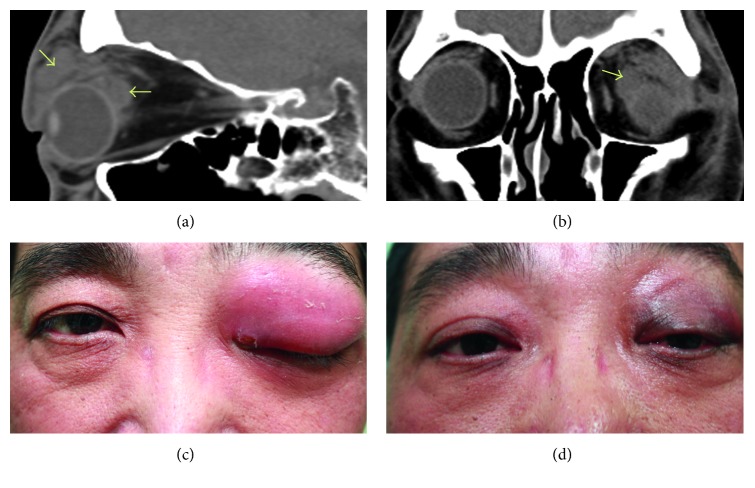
External eye photos and CT scan of case 6. (a, b) CT images. Orbital abscesses seen over the superior orbit and retrobulbar area (arrows). The sinuses were clear. (c) External eye photo before incision and drainage; (d) external photo 1 month after incision and drainage and antibiotic treatment.
